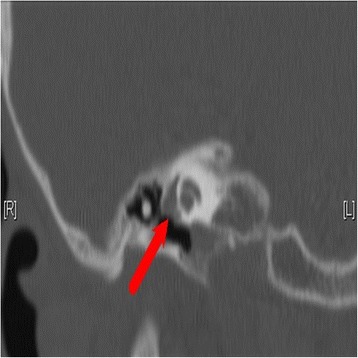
HRCT of the right side ear showed enlargement to facial nerve cannel (*red arrow*)
